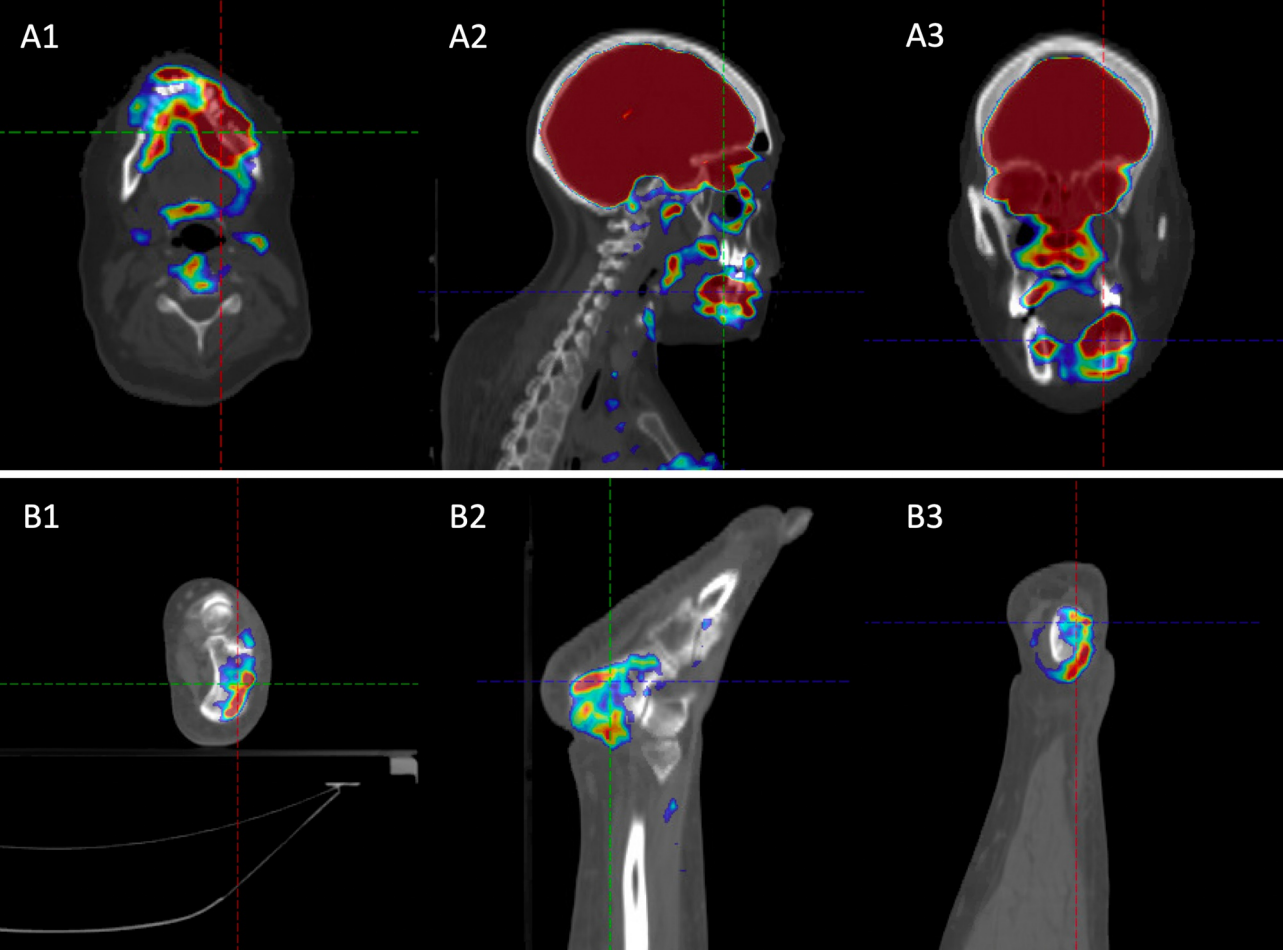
Representative images of positron emission tomography (PET)/CT at relapse, which shows the (A) mandibular and (B) right ankle 18-F fluorodeoxyglucose (FDG) avidity using an (1) axial view, (2) sagittal view, and (3) coronal view.
